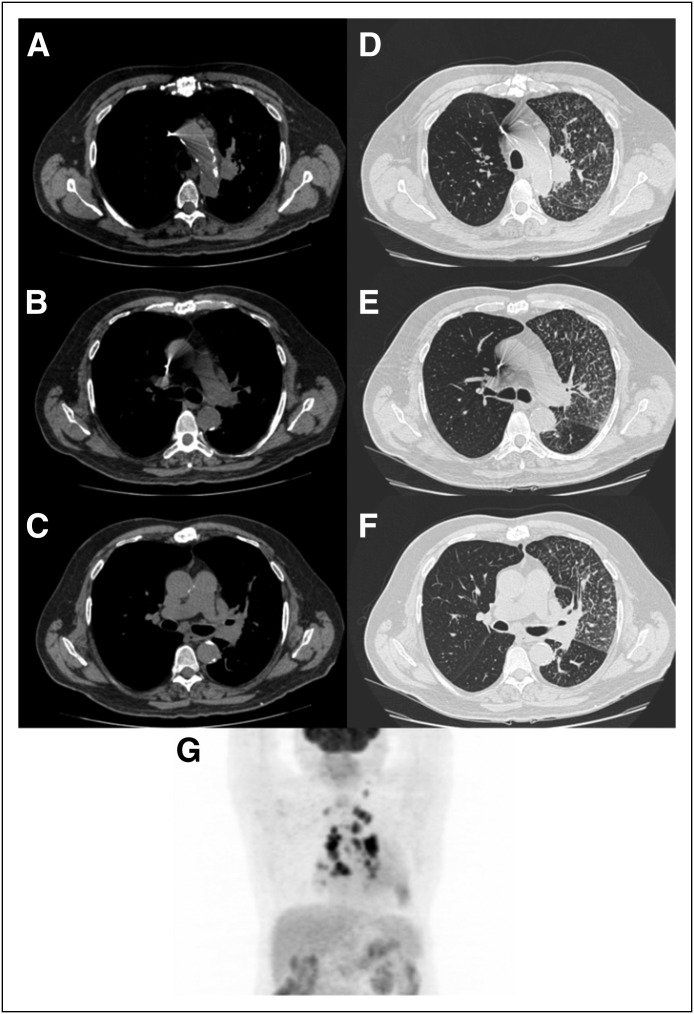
Computed tomography scan showing (A) left upper lung mass para aortic, (B)
